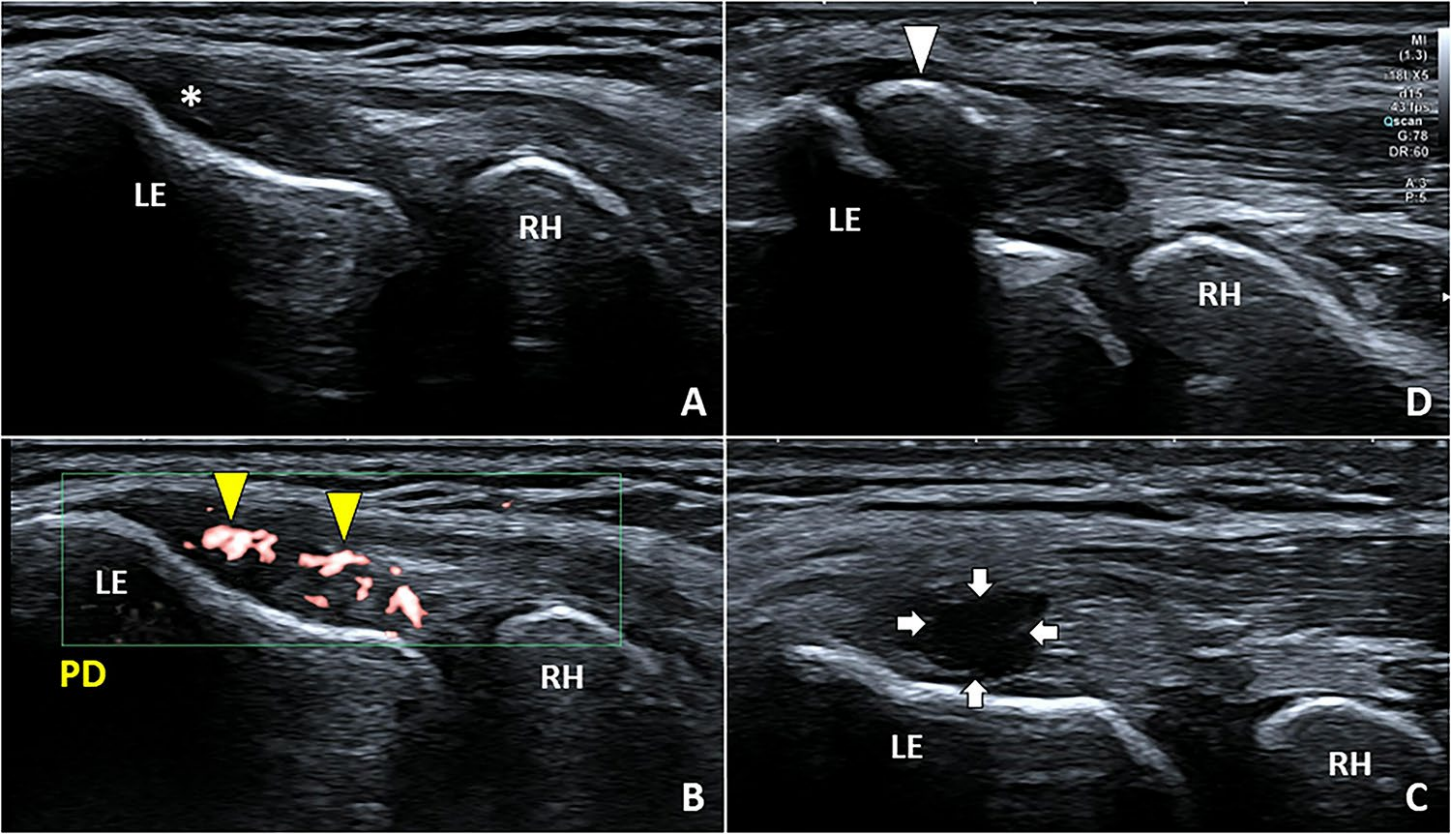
Hypoechoic thickening *(white asterisk)* of the CET in B-Mode (A) and neo-vessels *(yellow arrowheads)* in color/power Doppler (B) are the most common sonographic findings in padel players with lateral elbow tendinopathy. Focal injury *(white arrows)* of the CET (C) and calcific depositions *(white arrowheads)* can also be identified especially in players with recalcitrant lateral elbow pain (D). *LE* lateral epicondyle, *RH* radial head, *PD* power Doppler
